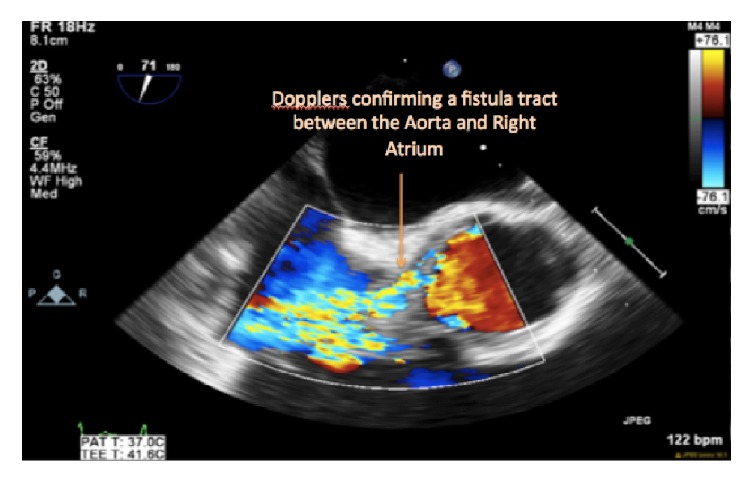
Axial image of the aortic root abscess with Doppler confirming the presence of a fistula between the aorta and the right atrium.
